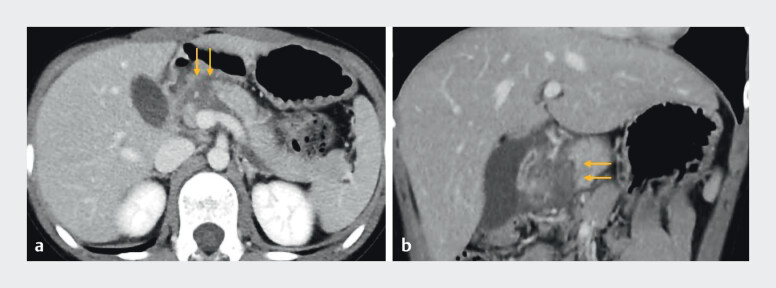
Computed tomography revealed disconnected pancreatic duct syndrome secondary to
